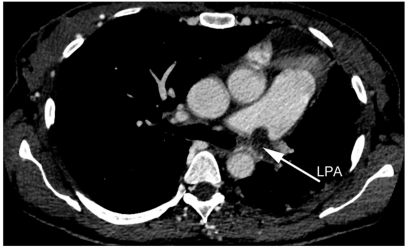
Total absence of the left lung perfusion in a 35-year-old female with severe pulmonary hypertension. Pulmonary computed tomography angiography (CTA) reveals complete occlusion of LPA with thrombus (arrow)
